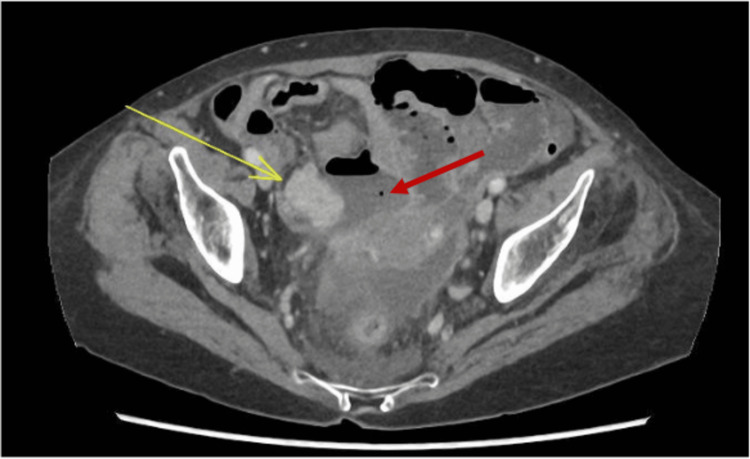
Contrast-enhanced computed tomography (CT) scan post perforation The yellow arrow indicates a homogeneously hyperenhancing mass (LMS) at the mid-distal ileum measuring approximately 31 x 20 mm in size (compared to 20mm in the previous scan) and the red arrow indicates gas and fluid-containing collection measuring approximately 43 x 49 mm.
